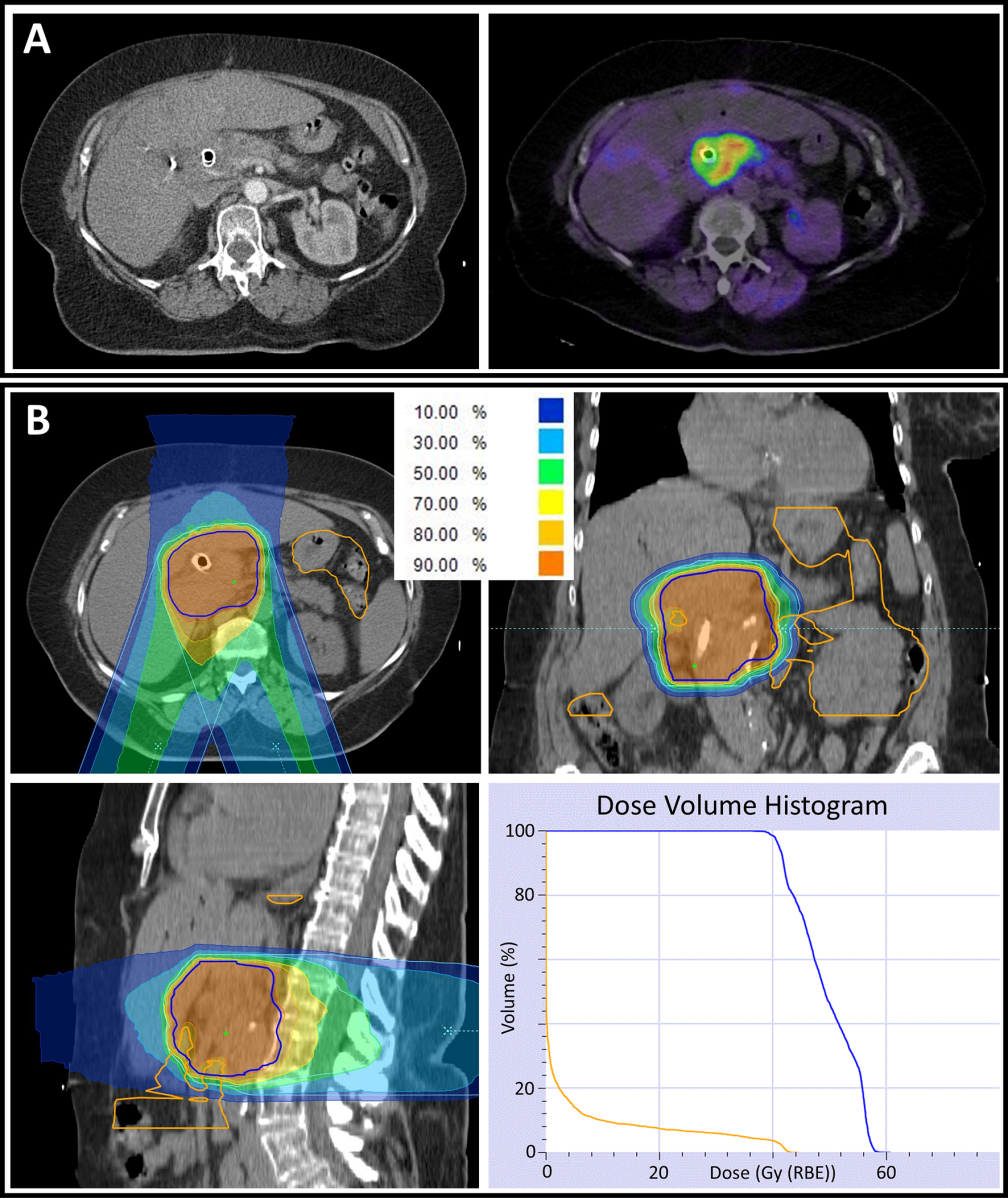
(A) Computed tomography (CT) scan of a locally advanced pancreatic cancer patient demonstrating a pancreatic tumor mass with an implanted biliary metal stent (left). To improve target volume definition in radiation planning, a fibroblast activation protein inhibitor-positron emission tomography (FAPI-PET) with a high tumor to background contrast was performed and matched with CT data (right). (B) Radiation plan of the same patient in axial (upper left), coronal (upper right) and sagittal (lower left) CT slices. The percentages of the different-colored isodose lines correspond to the prescribed dose of 48 Gy (RBE) in 12 fractions. For all volumes, an *α*/*β*-ratio of 2 Gy was used in the underlying relative biological effectiveness model. Partially, underdosage of the planning target volume (PTV, delineated in blue) needed to be accepted to respect the gastrointestinal (GI, delineated in orange) constraints. The dose volume histogram (lower right) demonstrates a ‘stereotactic-radiotherapy-like’ dose escalation within the PTV while respecting the GI constraints.
